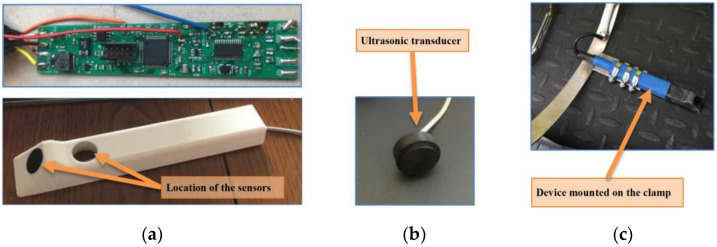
Photos of the electronic circuit (a top), digital printout of the device’s casing (a bottom), ultrasonic transducer (b), and device mounted on the clamp (c). Source: own.
